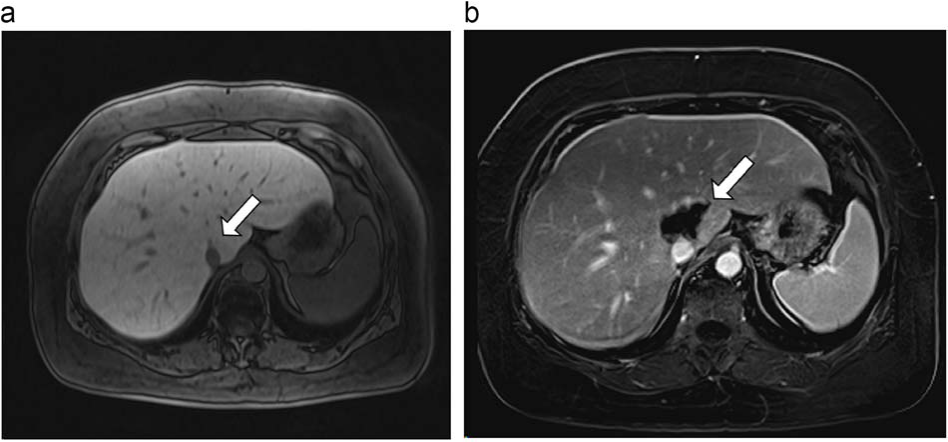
(a) Pre-ablation MRI demonstrates 6 mm lesion in caudate lobe of liver. (b) Post-ablation MRI (18 days postoperatively) demonstrates 40.4 mm complete ablation site in caudate lobe of liver.
